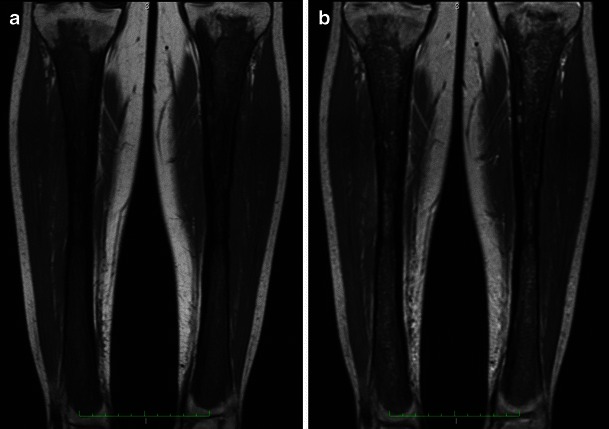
a Coronal T1-weighted spin-echo and b coronal T2-weghted spin-echo MR images of both legs in a 38-year-old female. There is symmetric low signal intensity of the diaphyses and metaphyses, sparing the epiphysis
